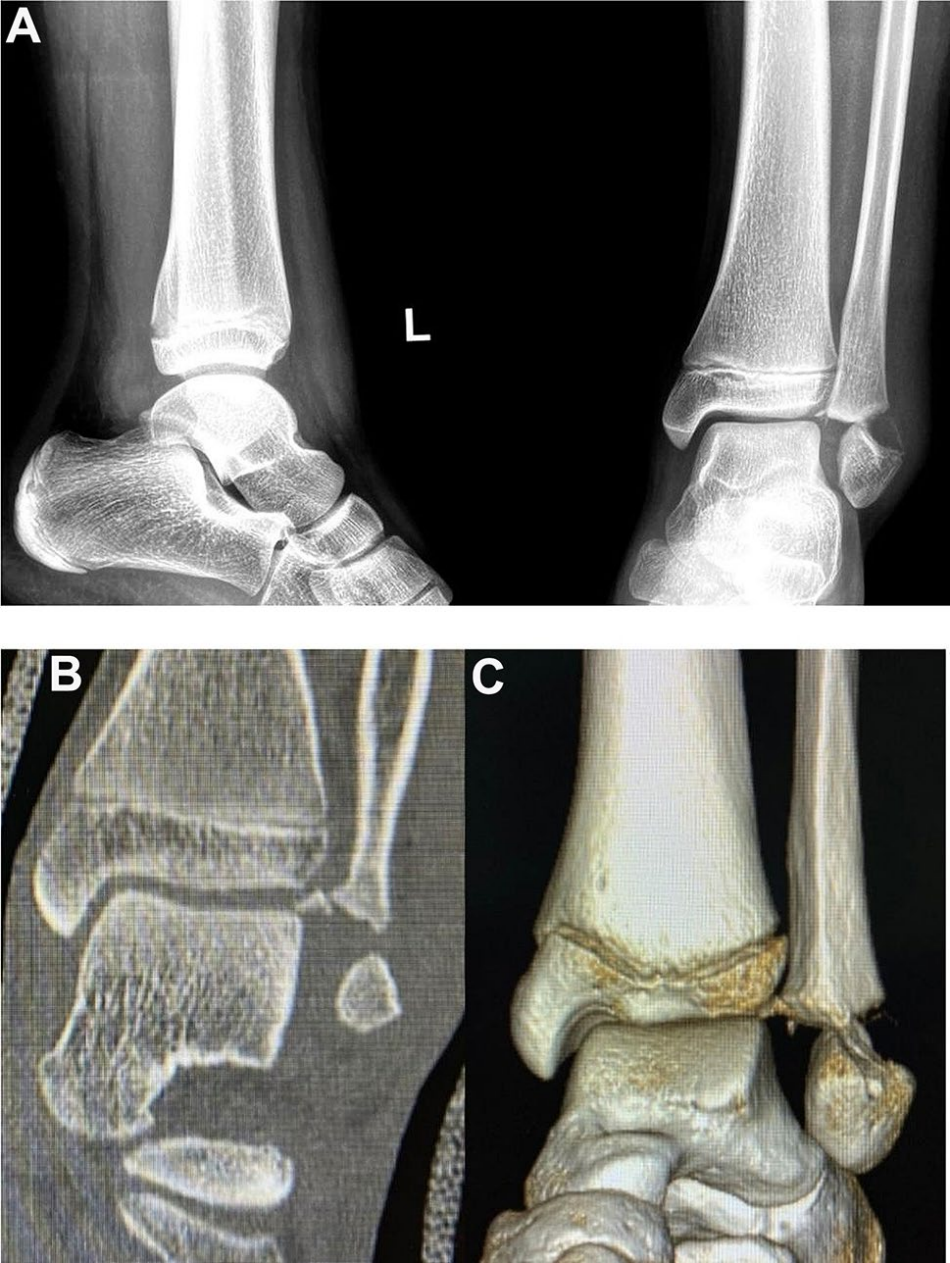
A: Standard AP and lateral radiographs taken on presentation to the emergency. B-C: Typical imaging of coronal CT and 3D images showing the fragment trapped in the syndesmosis
